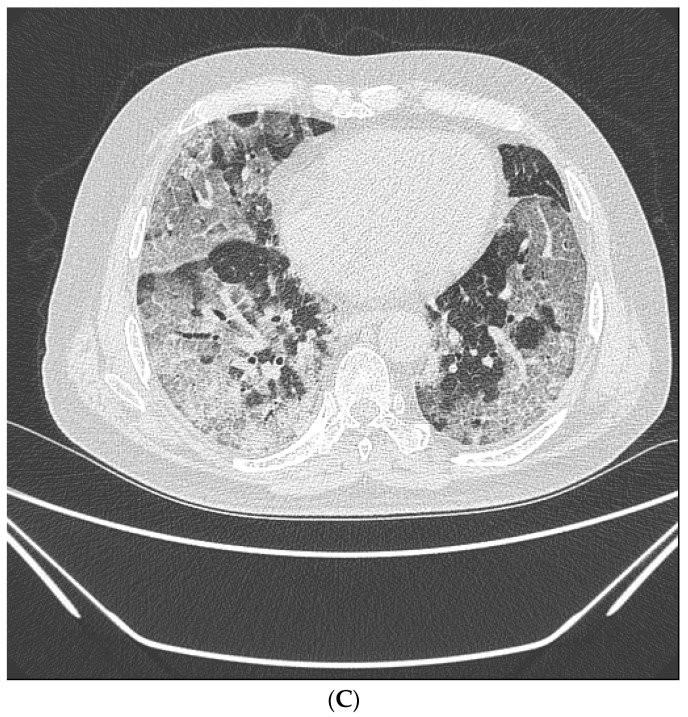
Typical findings for COVID-19 on CT scans. (A) Ground-glass opacity shows as a modest increase in lung attenuation on lung window CT images, not obscuring the pulmonary vessels. (B) Consolidation appears as high-density patchy opacities that obscure the margins of vessels and airway walls, inside which air bronchogram (arrow) could be observed. (C) Crazy-paving pattern appears as thickened interlobular septa and intralobular lines superimposed on a background of ground-glass opacity.
